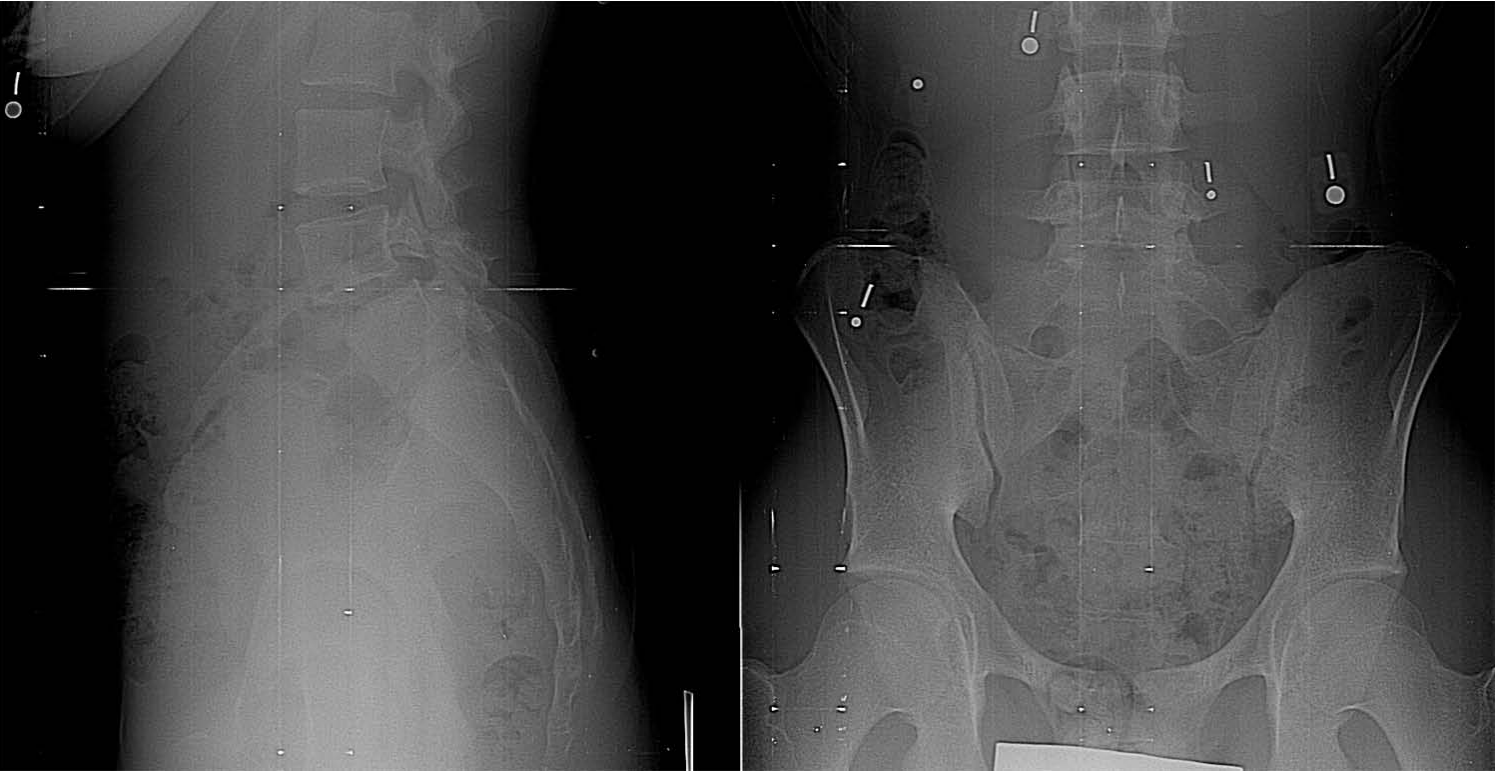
A case of low-grade spondylolisthesis for which there was complete intra- and inter-observer agreement among all observers (type I spondylolisthesis). Only the lumbosacral region from the original radiographs is provided.
